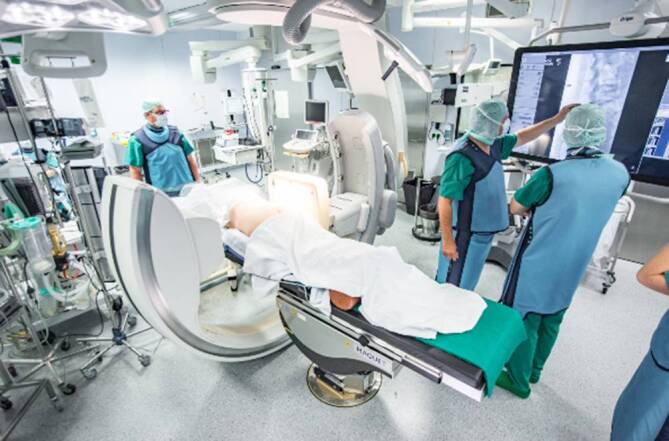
## Cone-beam-Computertomographie
